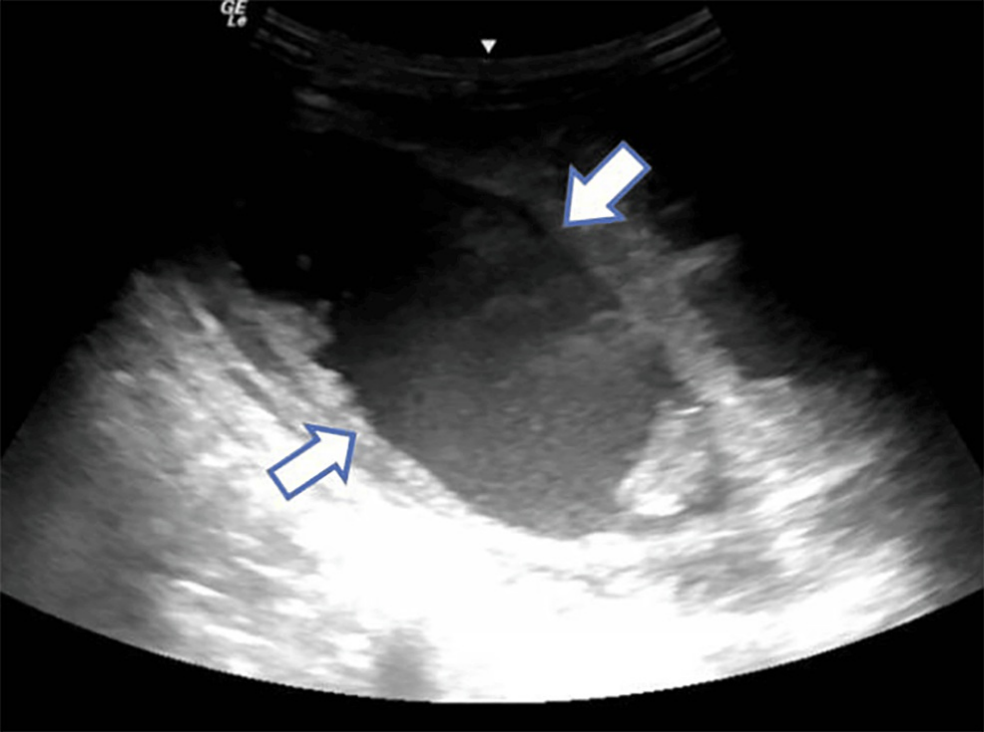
US evaluation of the right lower quadrant revealed a large subcutaneous collection (arrow) in the right lower quadrant, into which an 8-French percutaneous catheter was placed. US: Ultrasound
